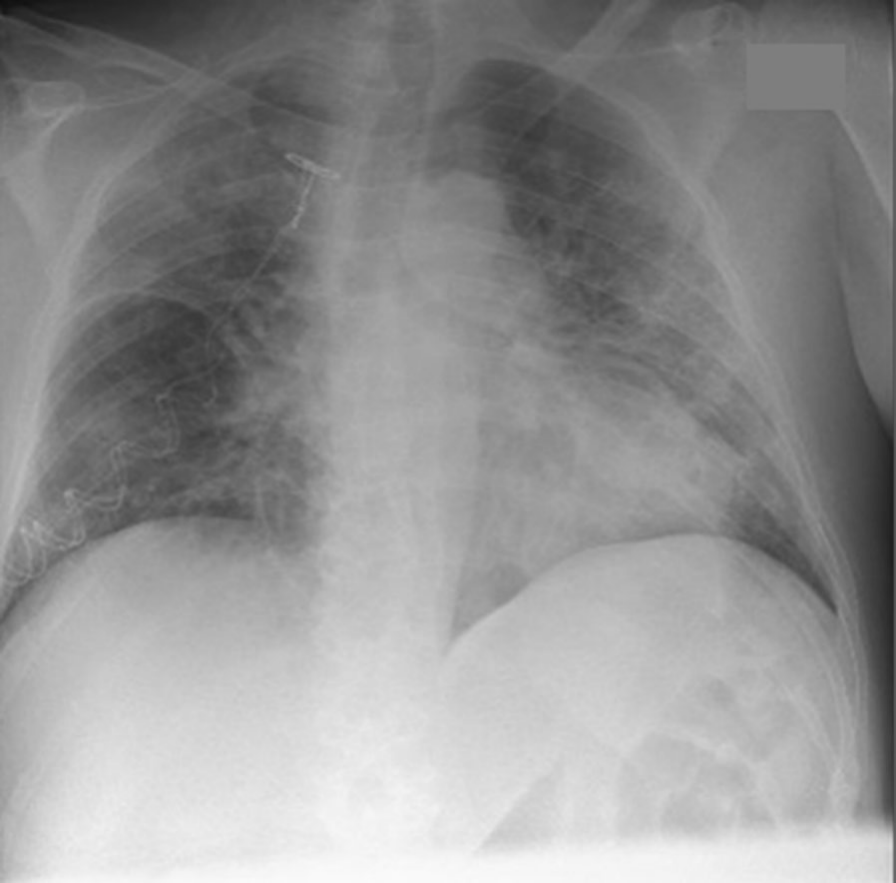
Chest X-ray obtained 1 day after readmission and diagnosis of SARS CoV-2 infection
